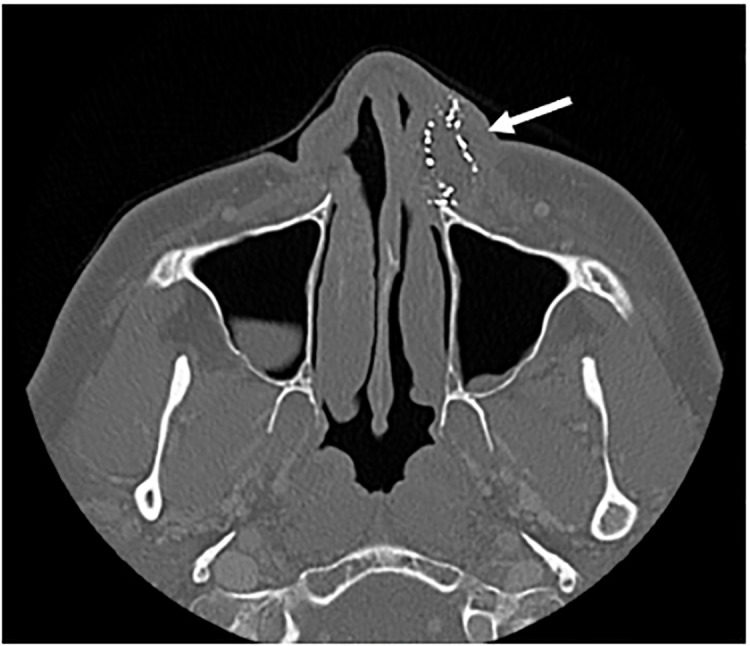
Computed tomography image of multiple embedded, high-density foreign bodies within left facial wound (arrow).
